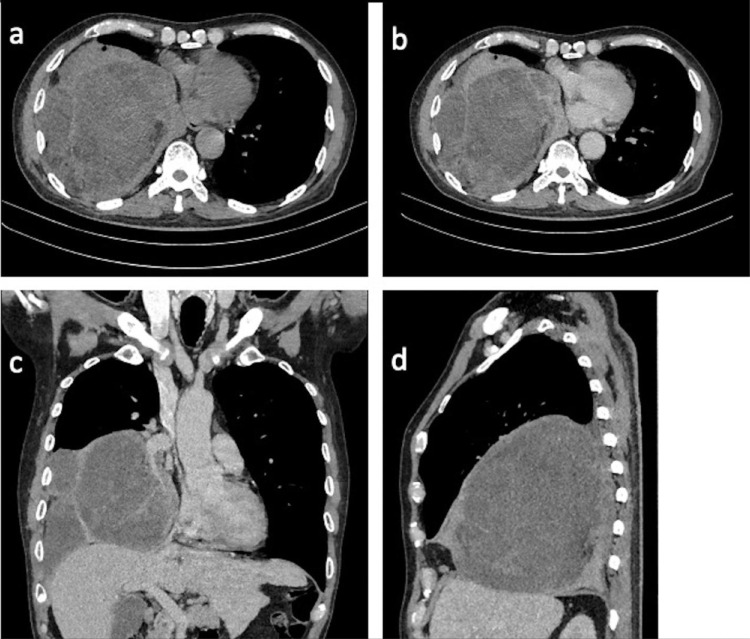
Chest CT, mediastinal window, unenhanced (a) and enhanced axial (b), coronal (c), and sagittal view (d). A solid mass (41 HU), measuring approximately 13.2 × 15.0 × 12.2 cm with fat and cystic component, clear margin, loculated, septated, with 4 mm septal thickness at the medial and lower lobe of the right lung (a). Contrast administration, mediastinal window, axial, coronal and sagittal view (b–d) showed contrast enhancement on solid and septated components (87 HU).
